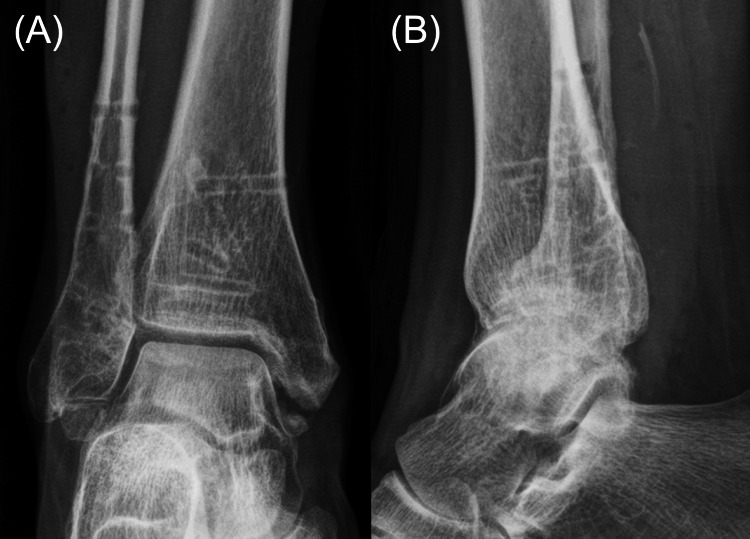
Plain radiographs of the right ankle in (A) anteroposterior and (A) lateral views at the 20-month final follow-up after fracture surgery demonstrating bone union without post-traumatic osteoarthritis
